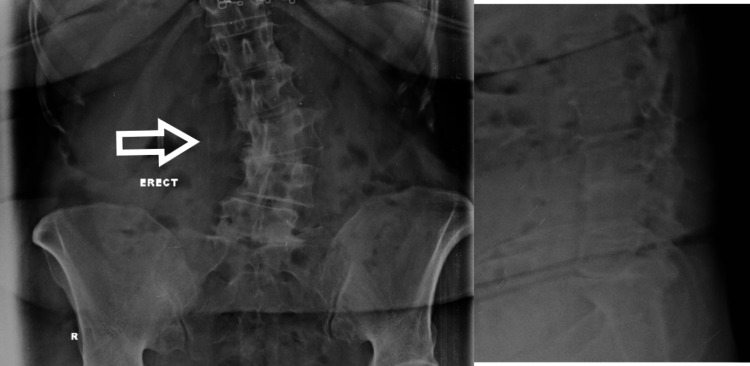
Pre-operative X-ray showing degenerative scoliosis
